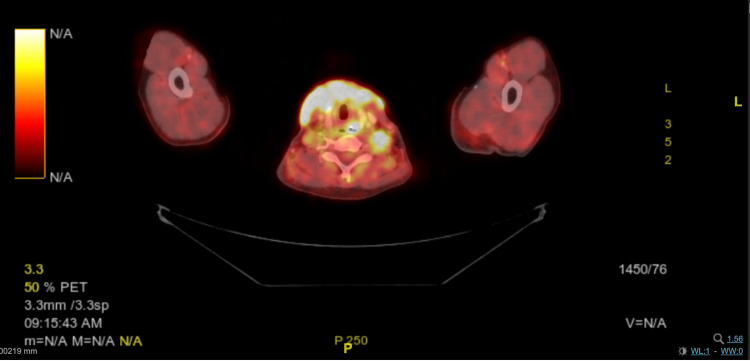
Fused image of FDG-18 PET CT showing diffuse uptake in the cutaneous region of the neck FDG-18 - Fluoro-deoxy-glucose-18; PET CT - Positron Emission Tomography-Computed Tomography
